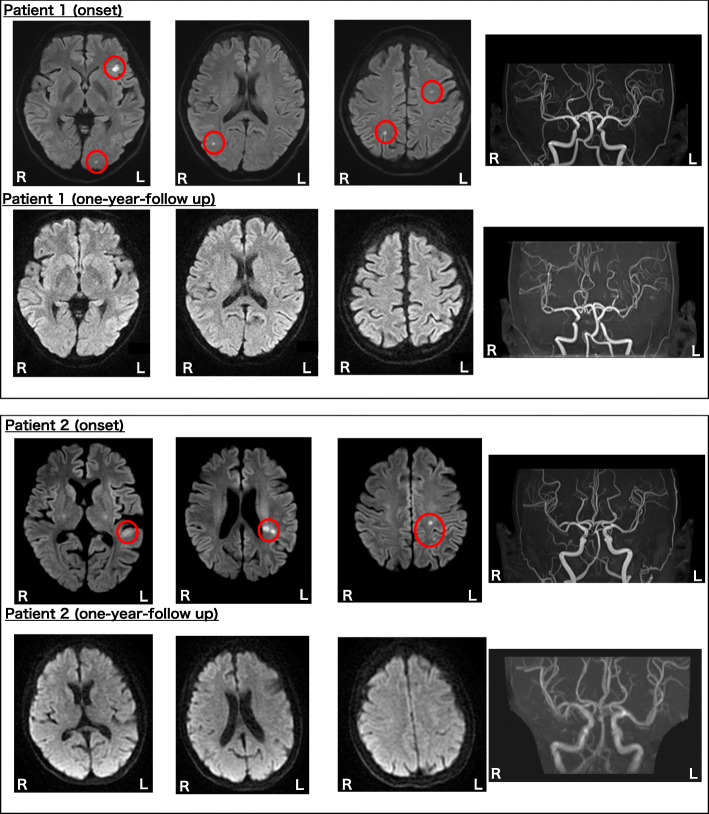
Brain MRI (Patient 1, 2). Patient 1 (Upper row) Brain diffusion-weighted MRI revealed disseminated cortical infarctions bilaterally. MR angiography showed no arterial stenosis. (Lower low) Brain diffusion-weighted MRI and MR angiography (one year after the discharge) revealed no findings. Patient 2 (Upper row) Brain diffusion-weighted MRI revealed focal brain infarction in the white matter underlying the left temporal transverse temporal gyrus, the left supramarginal gyrus, and the left parietal cortex. MR angiography showed no arterial stenosis. (Lower low) Brain diffusion-weighted MRI and MR angiography (one year after the discharge) revealed no findings
